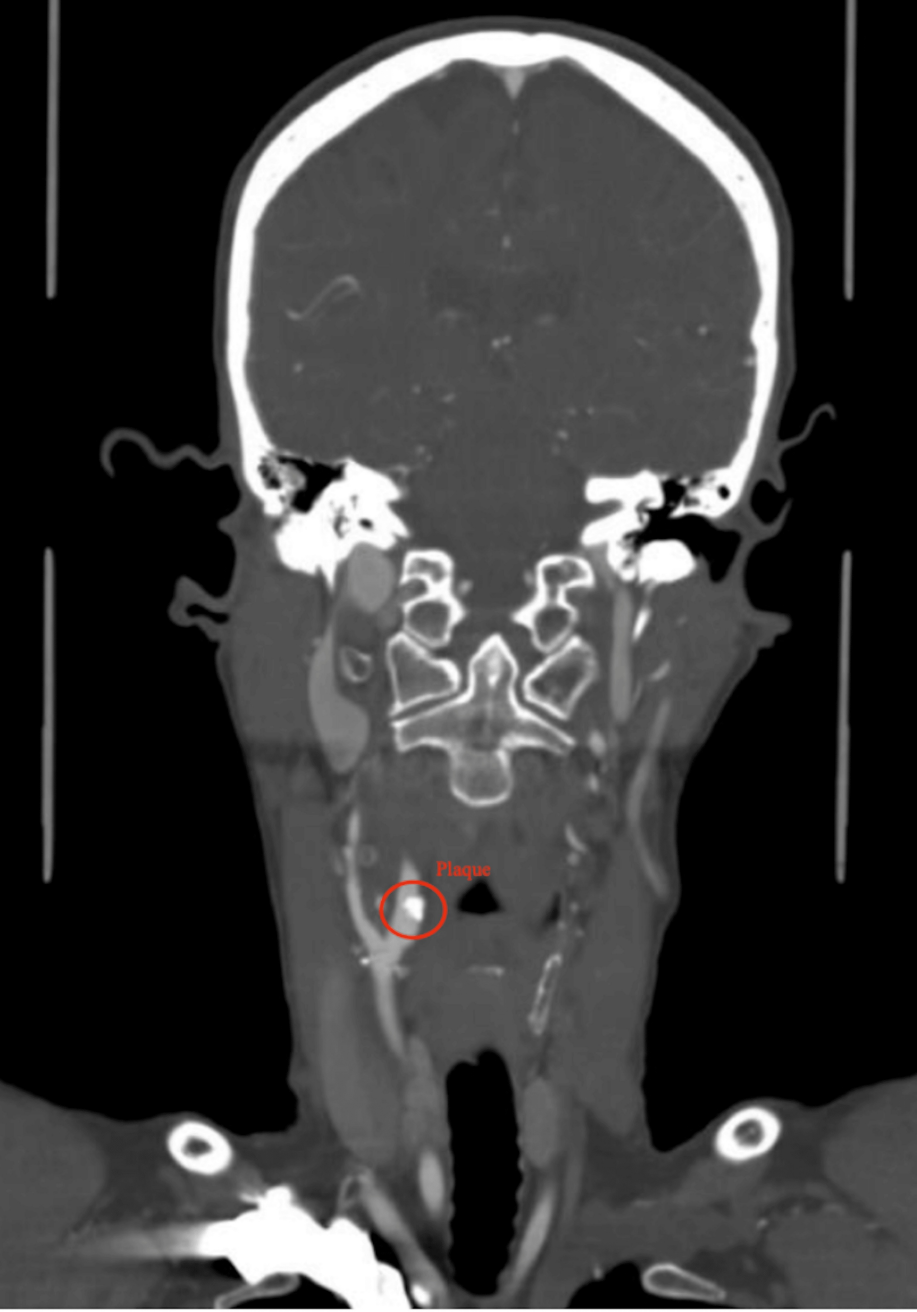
CT angiography showing a large right apical bulla (circled in red) CT, computed tomography
